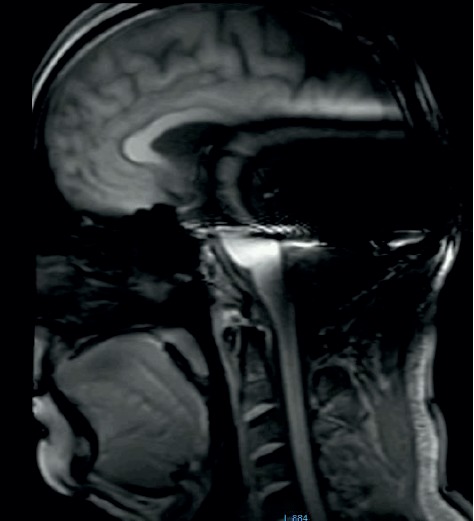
MRI survey pattern of the sagittal plain of a 3 T scanning of a Medel Synchrony Implant.
